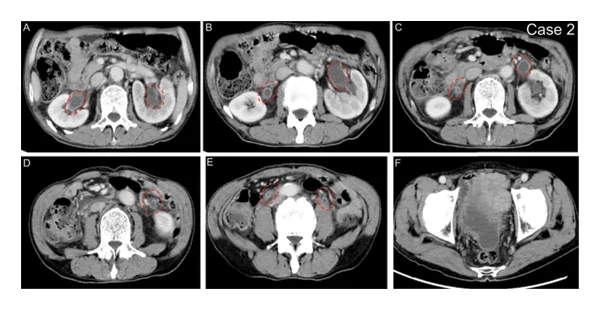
Case 2 patient’s CT scan revealed the inflammation of bilateral ureteral and bladder wall. The abdominal CT scan revealed mild dilatation of the bilateral renal pelves and ureters, accompanied by thickening of the ureteral walls. The bladder wall appears thickened with an irregular contour, suggesting an inflammatory process.
